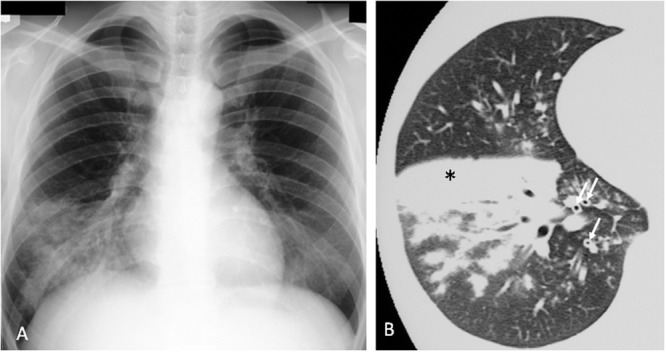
Mycoplasma pneumoniae* pneumonia in human. (A) Chest x-ray shows infiltrates in the right lower lobe. (B) Consolidation (^∗^) and bronchovascular bundles thickening (↑) on CT scan. Reproduced with permission from Tanaka and Hayashi (2007).
